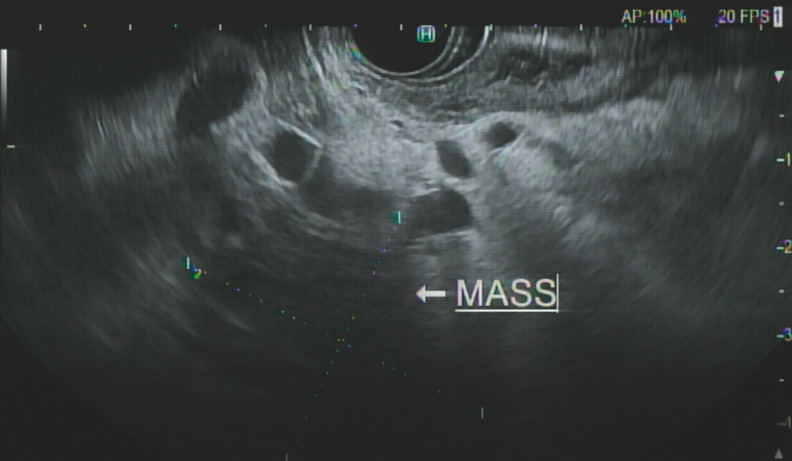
Endoscopic ultrasound demonstrating a large pancreatic mass.
